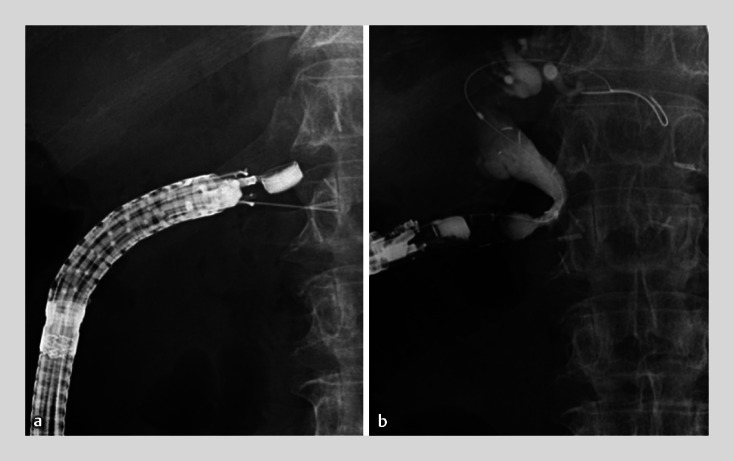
Endoscopic ultrasound-guided tissue acquisition followed by choledochoduodenostomy via the afferent limb.
